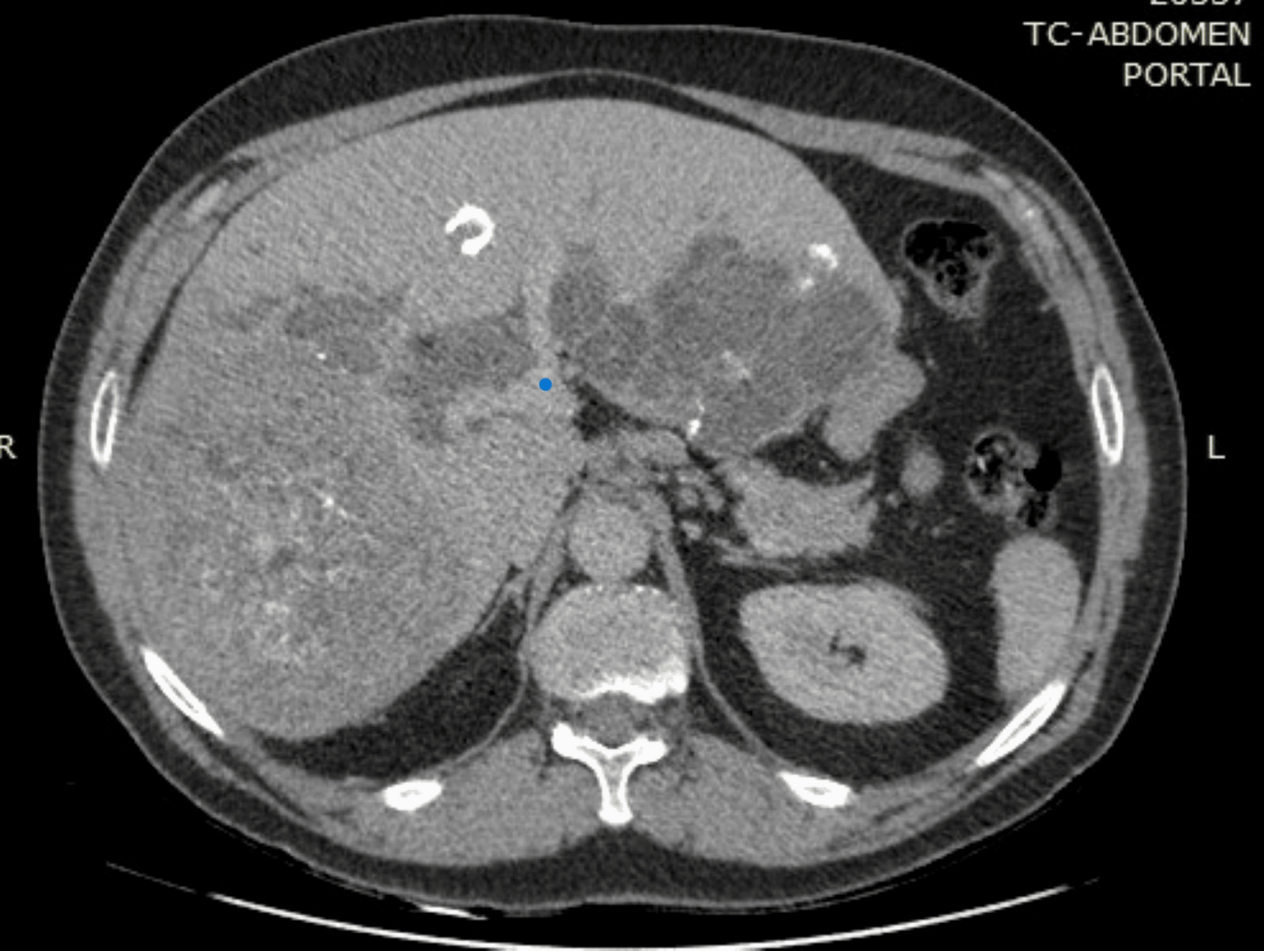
Computed tomography from March 2018 demonstrating multiple cystic lesions with typical coarse calcifications in both lobes, encompassing the portal vein (blue dot).
